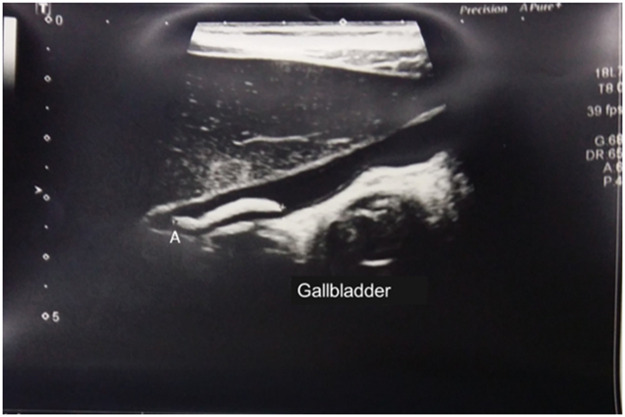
Biliary tract ultrasound. Note Ascaris in the gallbladder (A).
